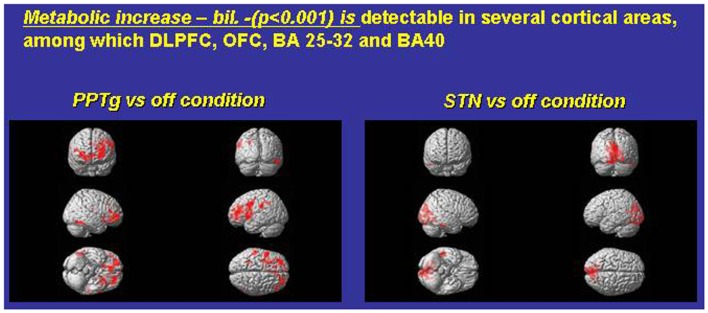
Exemplary FDG consumption comparing, in the same PD subjects, the condition *only* PPTg-ON (left panel) versus the condition STN-ON (imaging were performed in Pisa, under the supervision of Dr. Ceravolo and Dr. Volterrani; for details, see Ceravolo et al., 2011).
